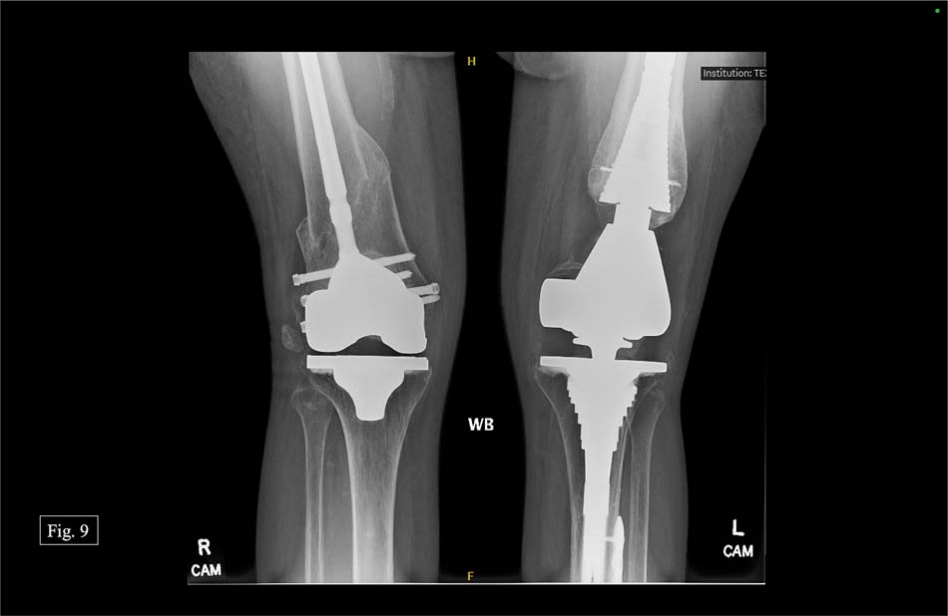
Bilateral, February 2024, AP X-ray.
